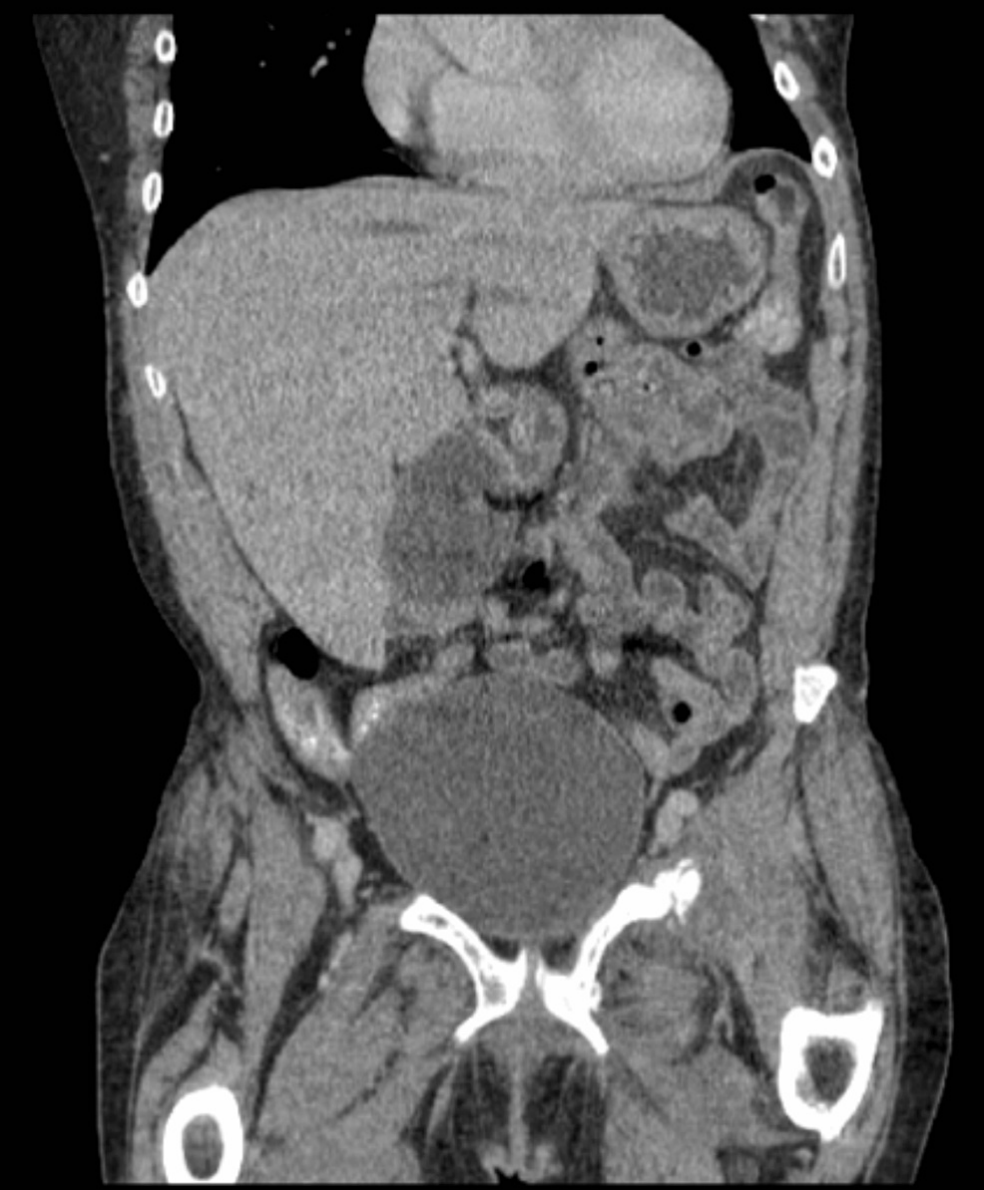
CT of the abdomen and pelvis This CT of the abdomen and pelvis with IV contrast obtained due to the patient's history of loose stool shows a normal liver. Gallbladder mildly distended but no wall thickening. Pancreas is atrophic, and the spleen is normal. Kidneys (not seen in the image) are normal.
